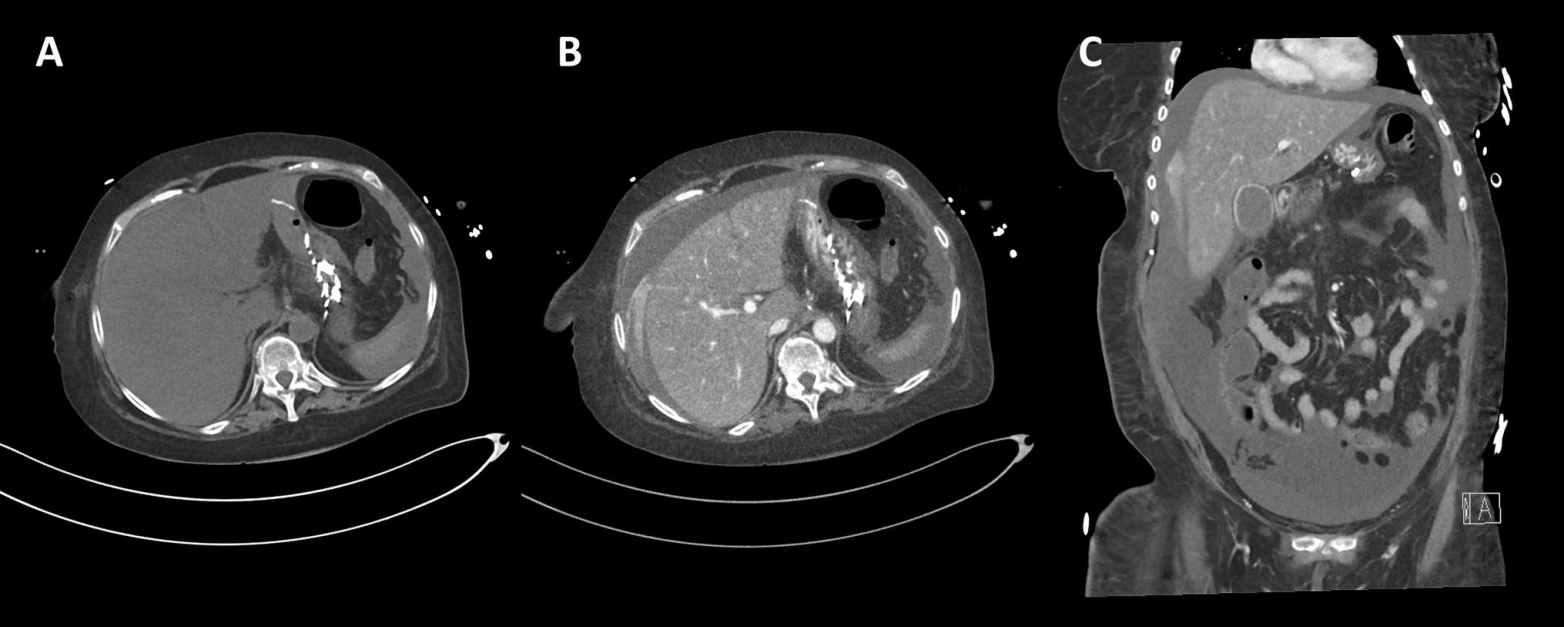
CT angiography of the abdomen (A native, axial plane, slice thickness 5 mm; B venous phase, axial, 1.5 mm; C venous phase, coronal, 3 mm). Hemorrhage originating from the liver capsule near segment six creates a contrast in the ascites. A small subcutaneous air pocket indicates the puncture site
